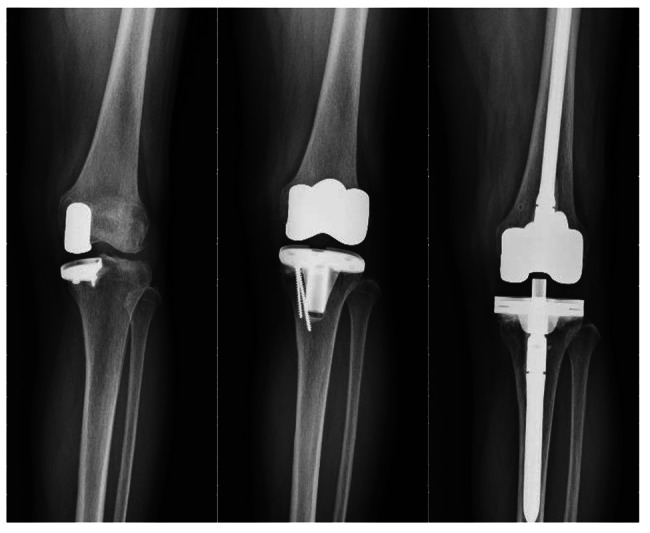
Anteroposterior radiograph of Patient A’s knee. Unicompartmental knee arthropasty (UKA) was performed in the past (left), procedure with primary prostheses and bone graft after UKA failure (center), and procedure with revision prostheses after primary prostheses failure due to tibia site loosening (right)
